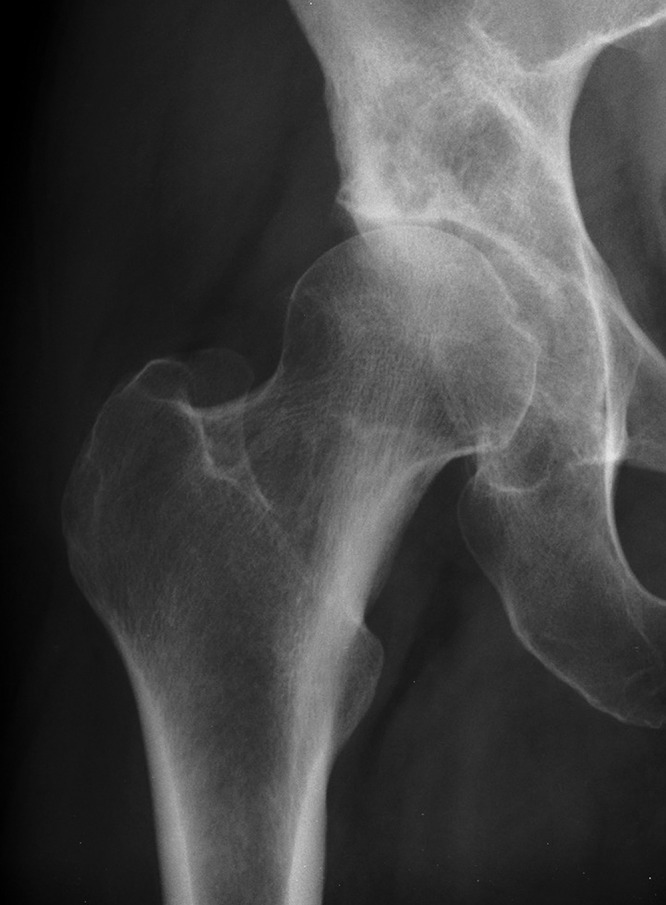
Anteroposterior x-ray of right hip with a superiorly located acetabular cyst
